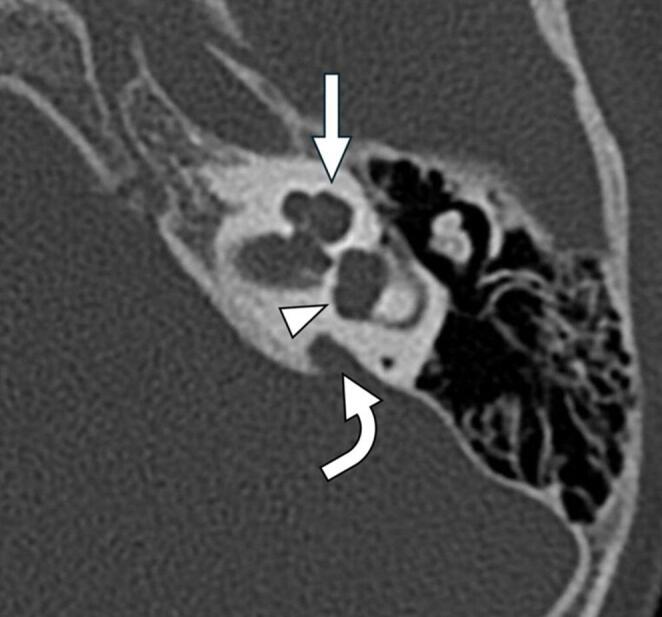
Incomplete partition type II. Axial CT showing a cystic cochlear apex (arrow), enlarged vestibule (arrowhead), and dilated vestibular aqueduct (curved arrow), consistent with incomplete partition type II
